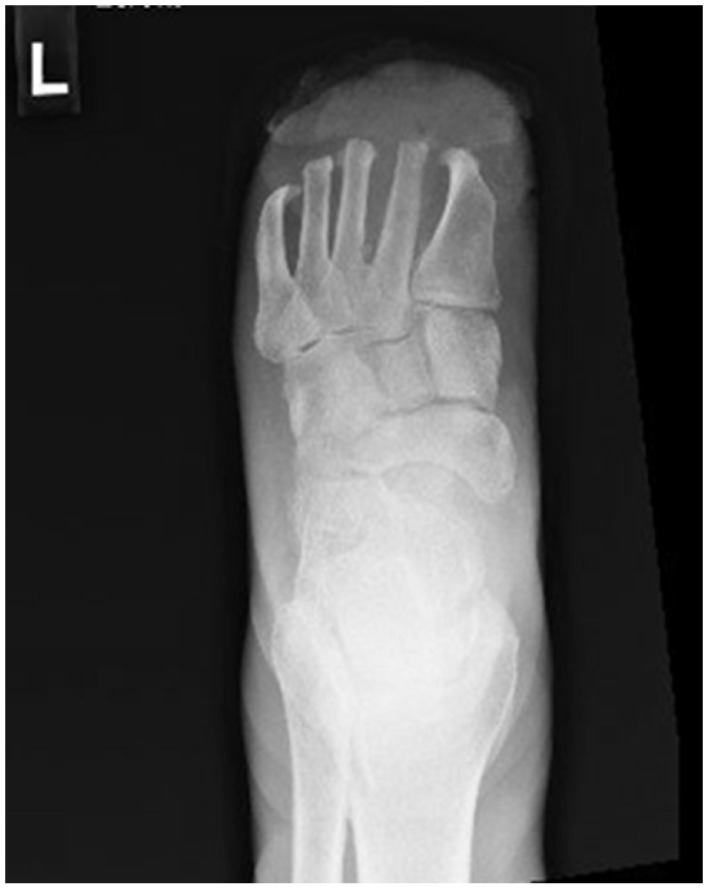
Left foot radiograph with soft tissue ulceration and periosteal reaction of the distal metatarsal shafts.
